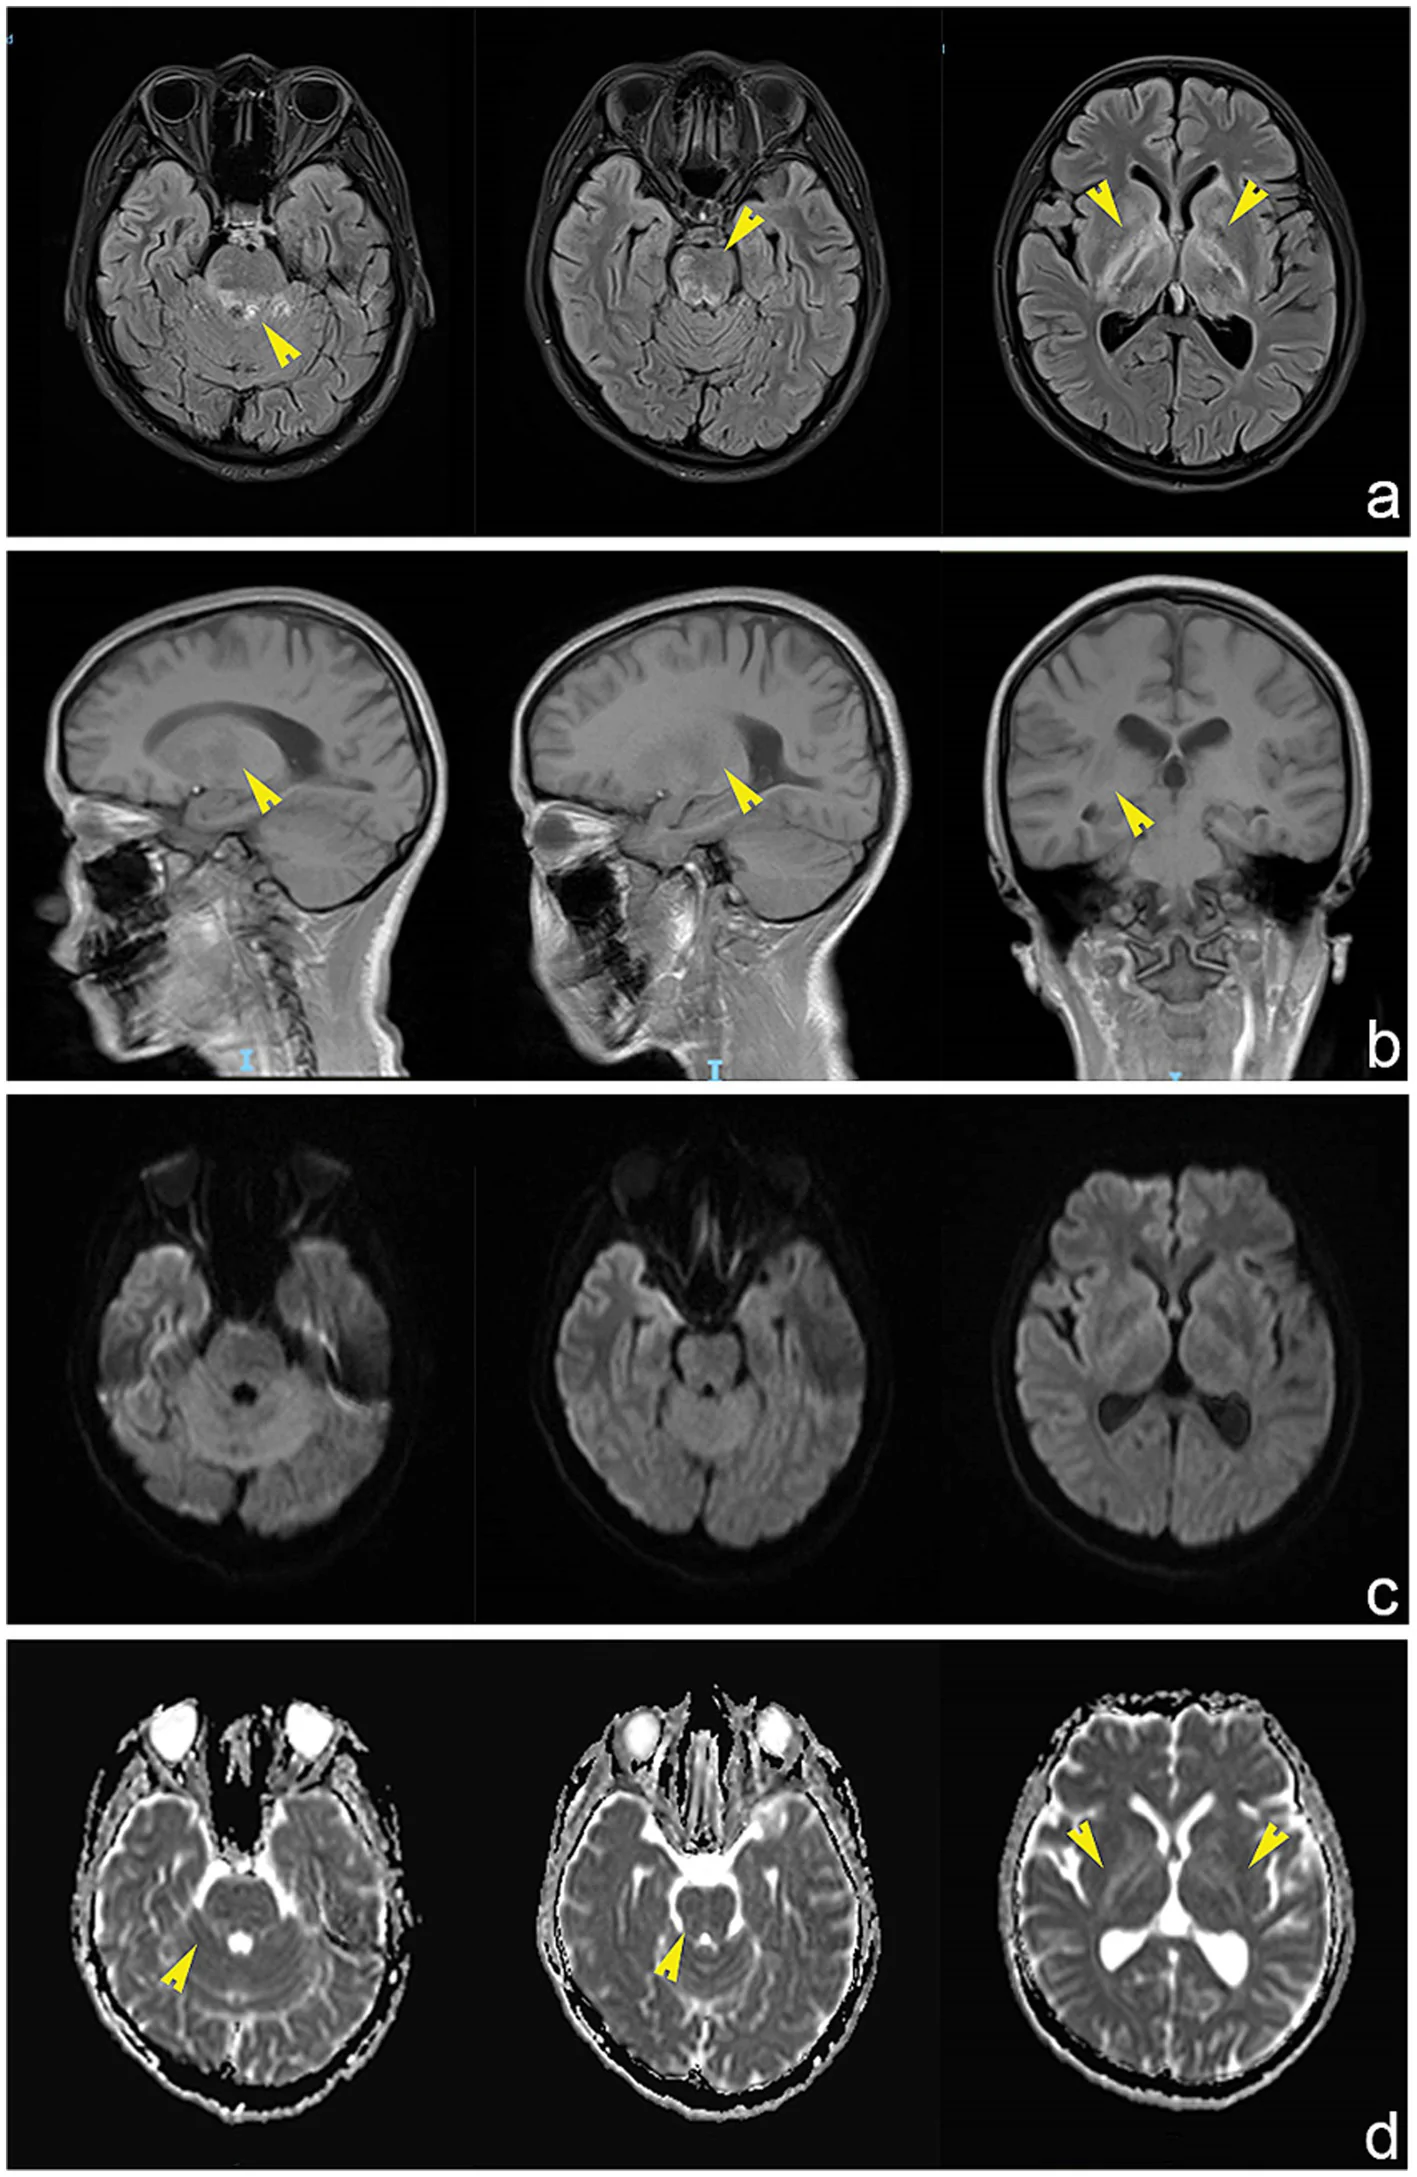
Brain MRI of the patient. (a) FLAIR sequences revealed multiple hyperintense lesions (yellow arrows) involving the pons, bilateral middle cerebellar peduncles, bilateral thalami, and bilateral basal ganglia. The lesions were hypointense on T1WI (b) and appeared isointense on DWI images (c) and hyperintense on ADC maps (d).
